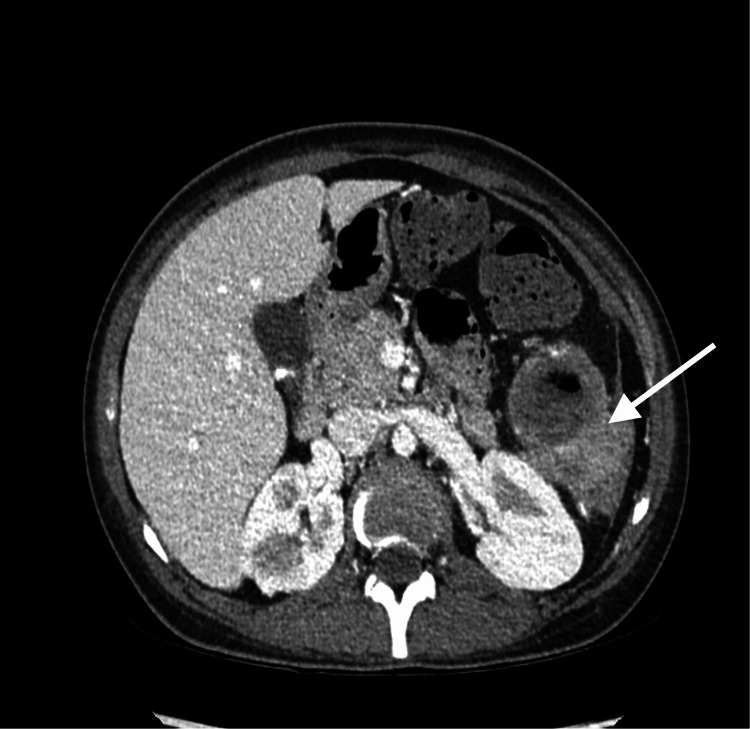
CT scan of the abdomen showing a mild thickening of the adjacent peritoneal reflection at the lower pole of the spleen (white arrow).
